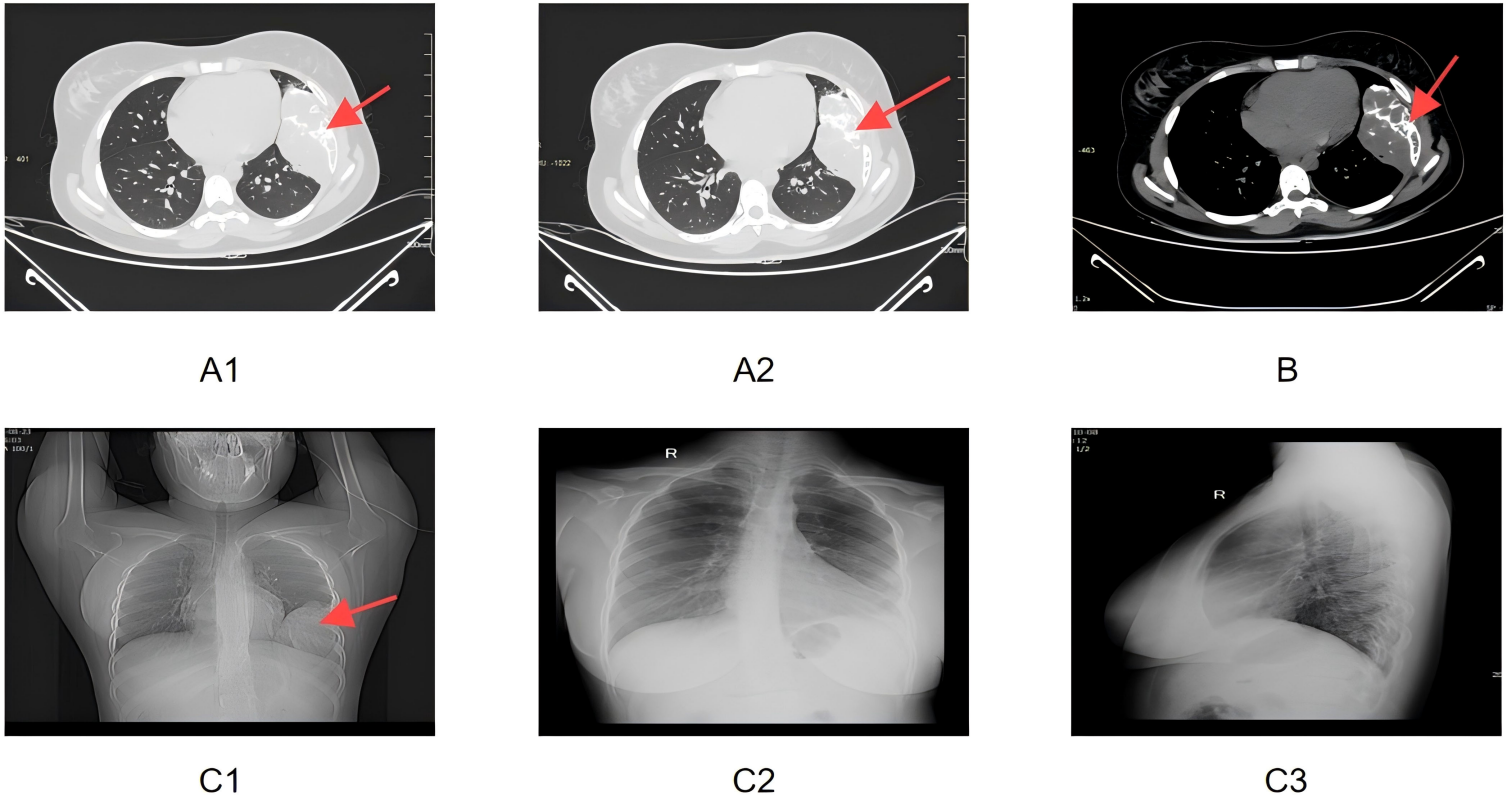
(A1, A2) Enhanced CT lung window images showing the expansile, osteolytic destruction of the left 6th rib, accompanied by soft tissue mass formation. Multiple patchy and arc-like calcifications and internal septations are visible. The red arrows point to the area of the rib destruction and the soft tissue mass. (B) Enhanced CT mediastinal window image demonstrating destruction of the left 6th rib and associated soft tissue mass, with heterogeneous enhancement. The red arrow highlights the region of the rib damage and the mass’s heterogeneity. (C1) Preoperative bedside frontal chest X-ray showing symmetrical thoracic structures, centered trachea and mediastinum, and expansile destruction of the left 6th rib, with soft tissue swelling and increased lung markings. The red arrow indicates the expansile destruction area of the 6th rib. (C2, C3) Postoperative bedside frontal and lateral chest X-rays showing partial absence of the left 6th rib, chest tube in the left pleural cavity, and soft tissue swelling with gas in the left chest wall as postoperative changes.
